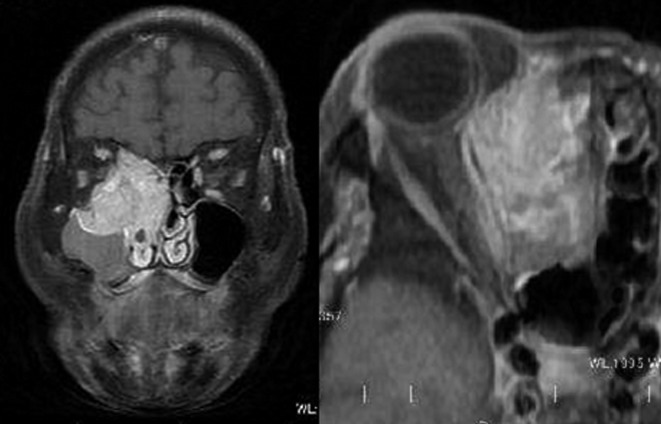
MRI showing a right-sided maxillary sinus tumor invading into the dura mater and orbit. MRI, magnetic resonance imaging.
